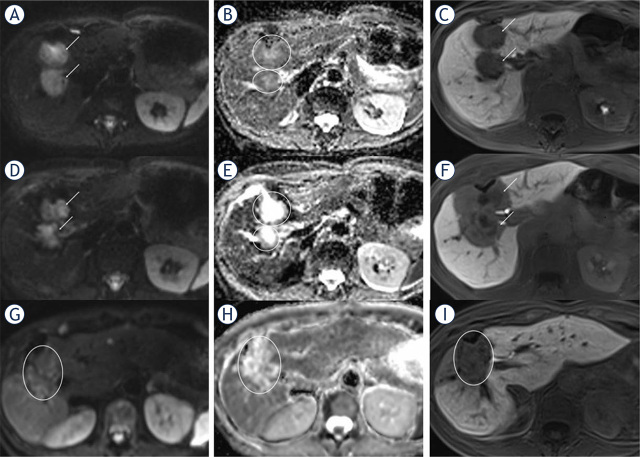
R-Met in a 62-year-old female. The pre-interventional diffusion-weighted imaging (DWI) shows two diffusion-restricted liver metastases with high signal on axial diffusion-weighted (DW)-MR image b = 800 s/mm^2^
